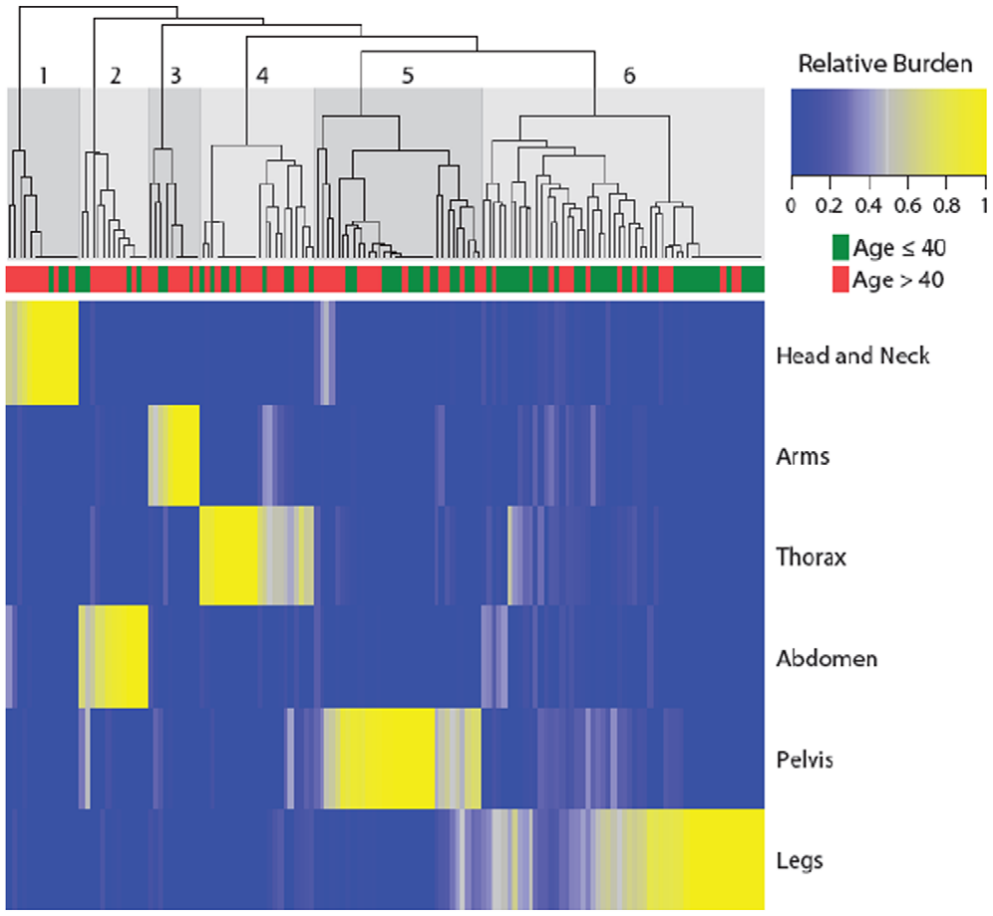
Results of unsupervised hierarchical clustering of patients based on relative tumor volume across body regions. (This figure is an interactive display. It is currently available for at www.wholebodymri.org). Unsupervised hierarchical clustering of relative tumor volume across body region (head/neck, trunk, extremities) for 145 subjects with internal nerve sheath tumors. Total tumor volumes were combined and scaled for each patient. Regions with higher tumor burden are shown in yellow. Individual patients are represented as columns and the maximum intensity projection (MIP) of the whole-body MRI scan is shown to the right of the clustering figure. For each patient, the Gini coefficient is shown in the lower left and the whole-body tumor volume in the lower middle.
